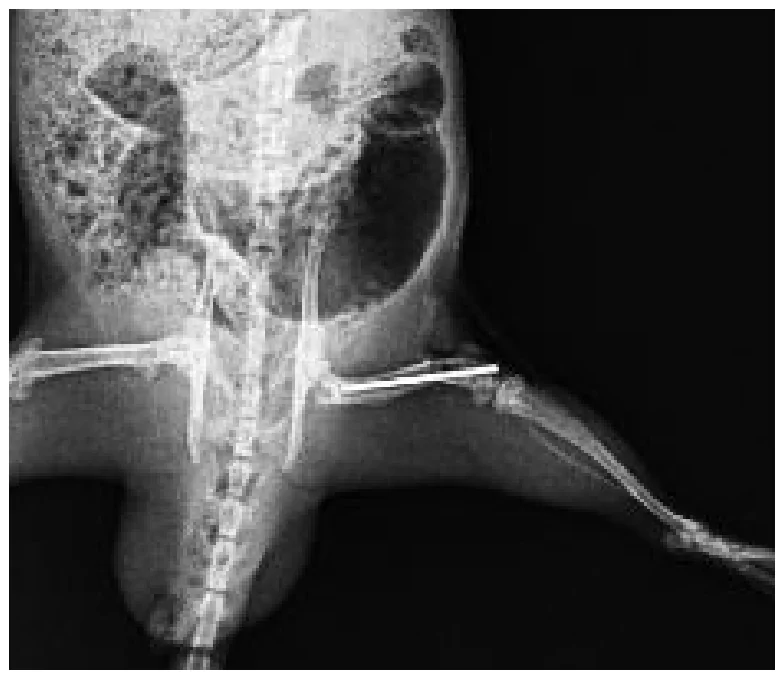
Representative anteroposterior radiograph obtained immediately after surgery demonstrating a standardized transverse fracture of the right femoral mid-diaphysis stabilized with an intramedullary Kirschner wire (K-wire). The radiograph was obtained to confirm successful fracture creation and appropriate intramedullary fixation.
